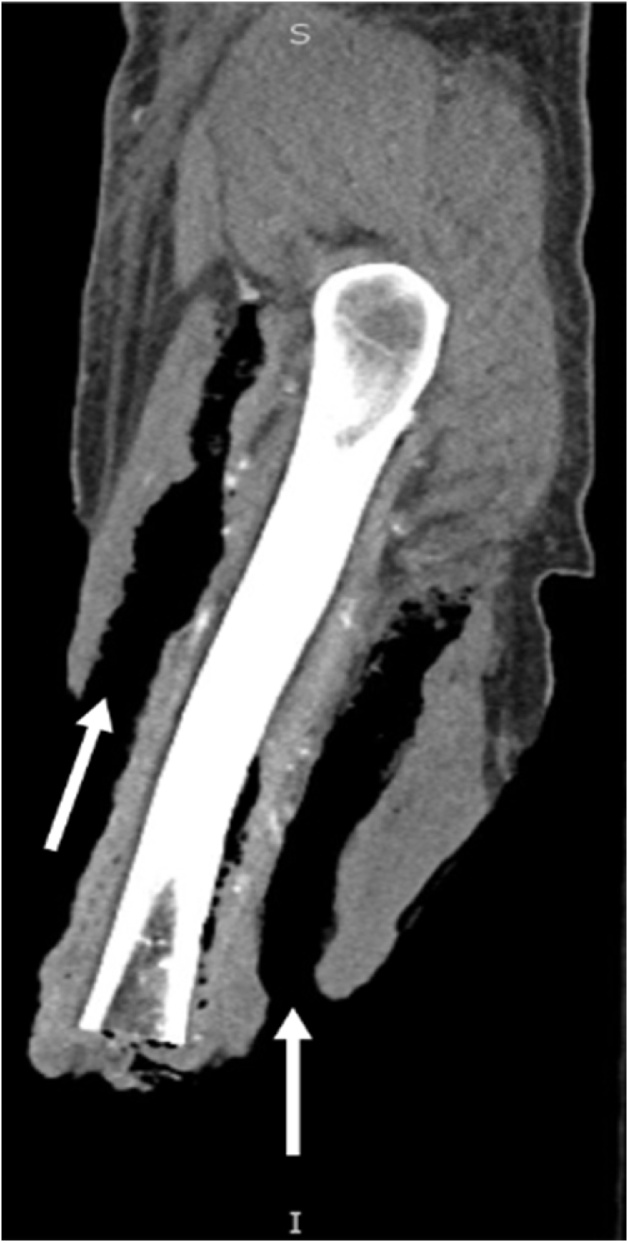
CT scan of right lower extremity. Extensive air is shown tracking superiorly within the anterior and posterior compartments of the thigh (notated by white arrows).
